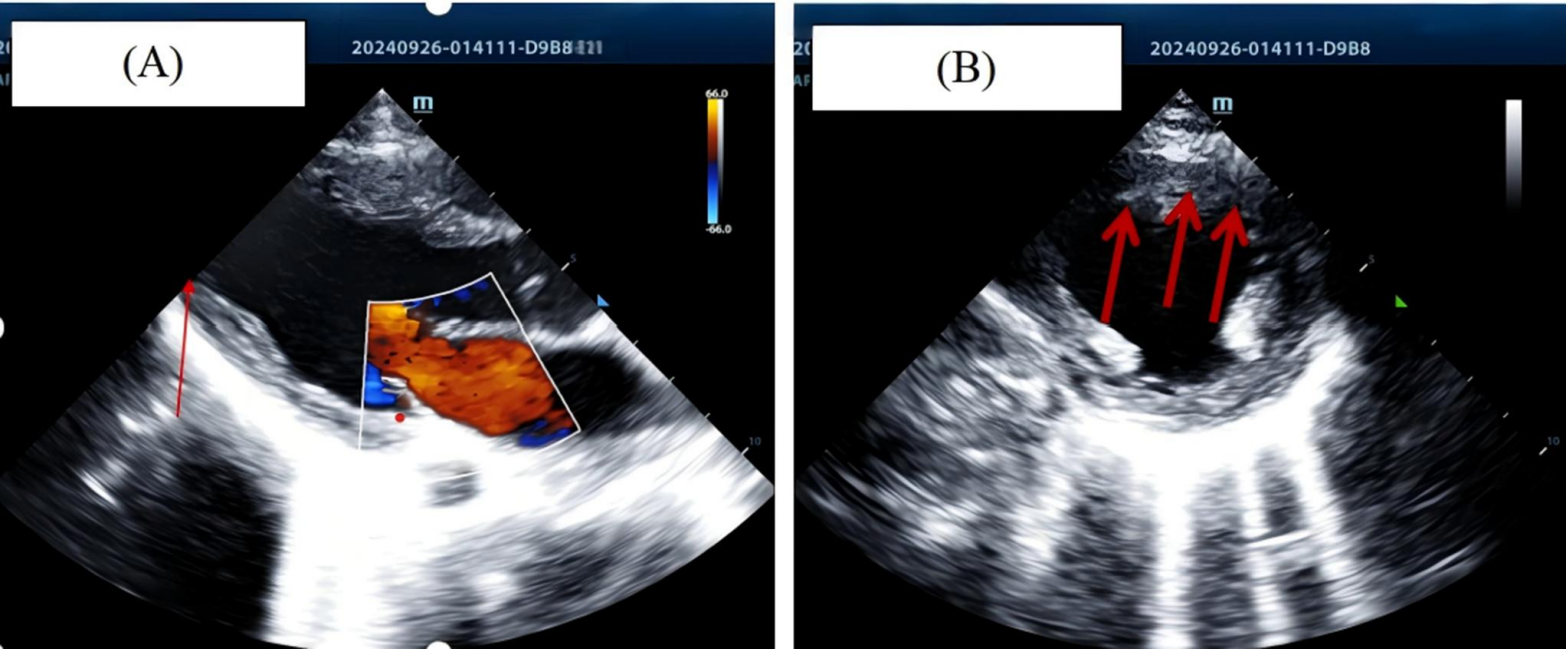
(A) short-axis view showing hypokinesis of the anterior left ventricular wall and apical segments. (B) Apical four-chamber view showing hypokinesis of the anterior left ventricular wall and apical segments.
